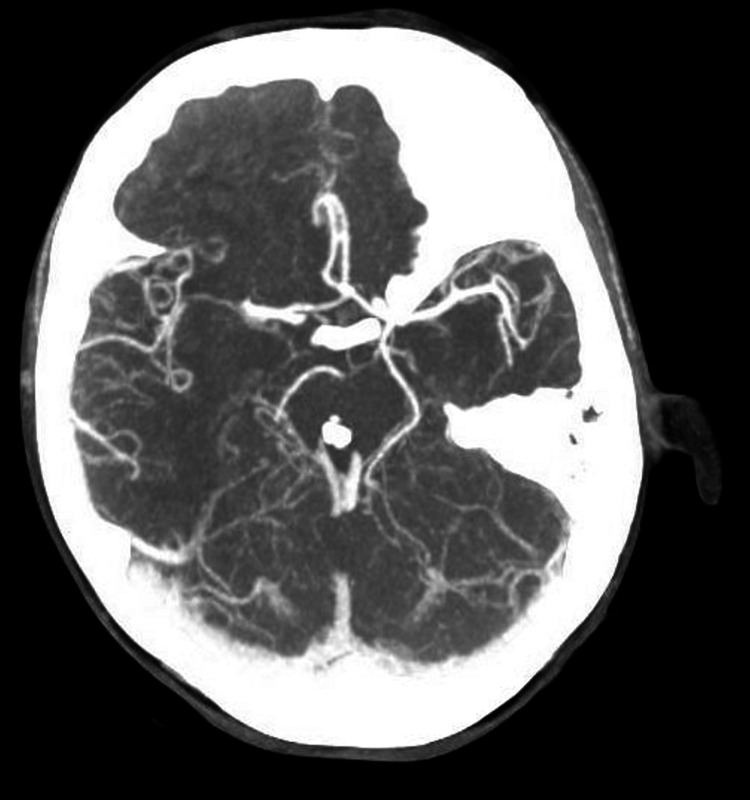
CT angiography of the head showing no significant arterial stenosis, arterial occlusion, intracranial aneurysm, or AVMs AVM: Arteriovenous malformations
